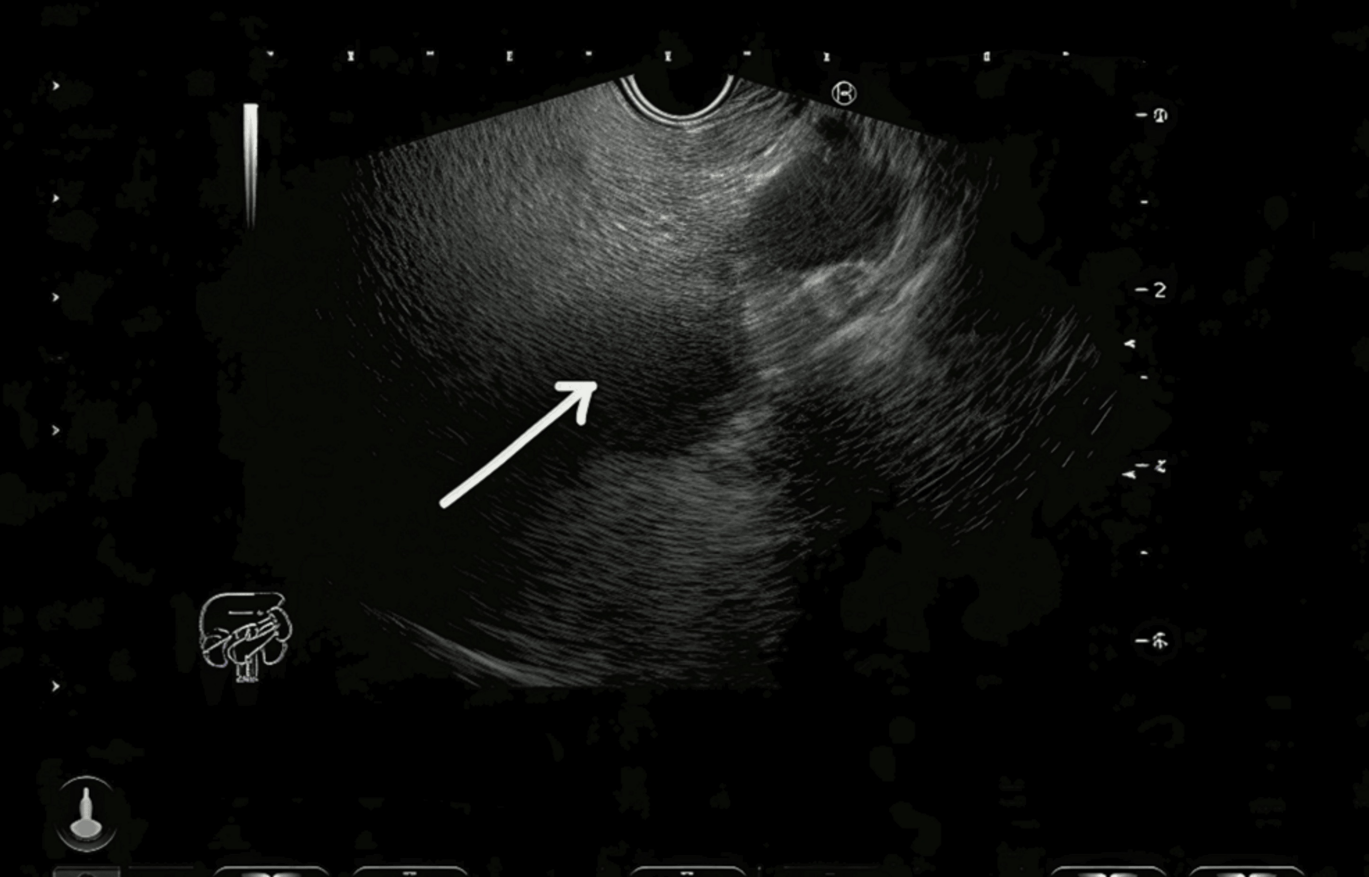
EUS showing a dilated distal common bile duct (CBD) remnant. EUS, endoscopic ultrasound
